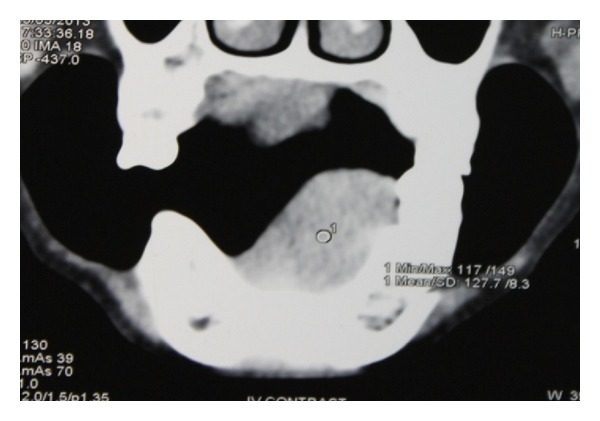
Axial CT shows lingual nerve (small circle) found within the mass in the left floor of the mouth.
